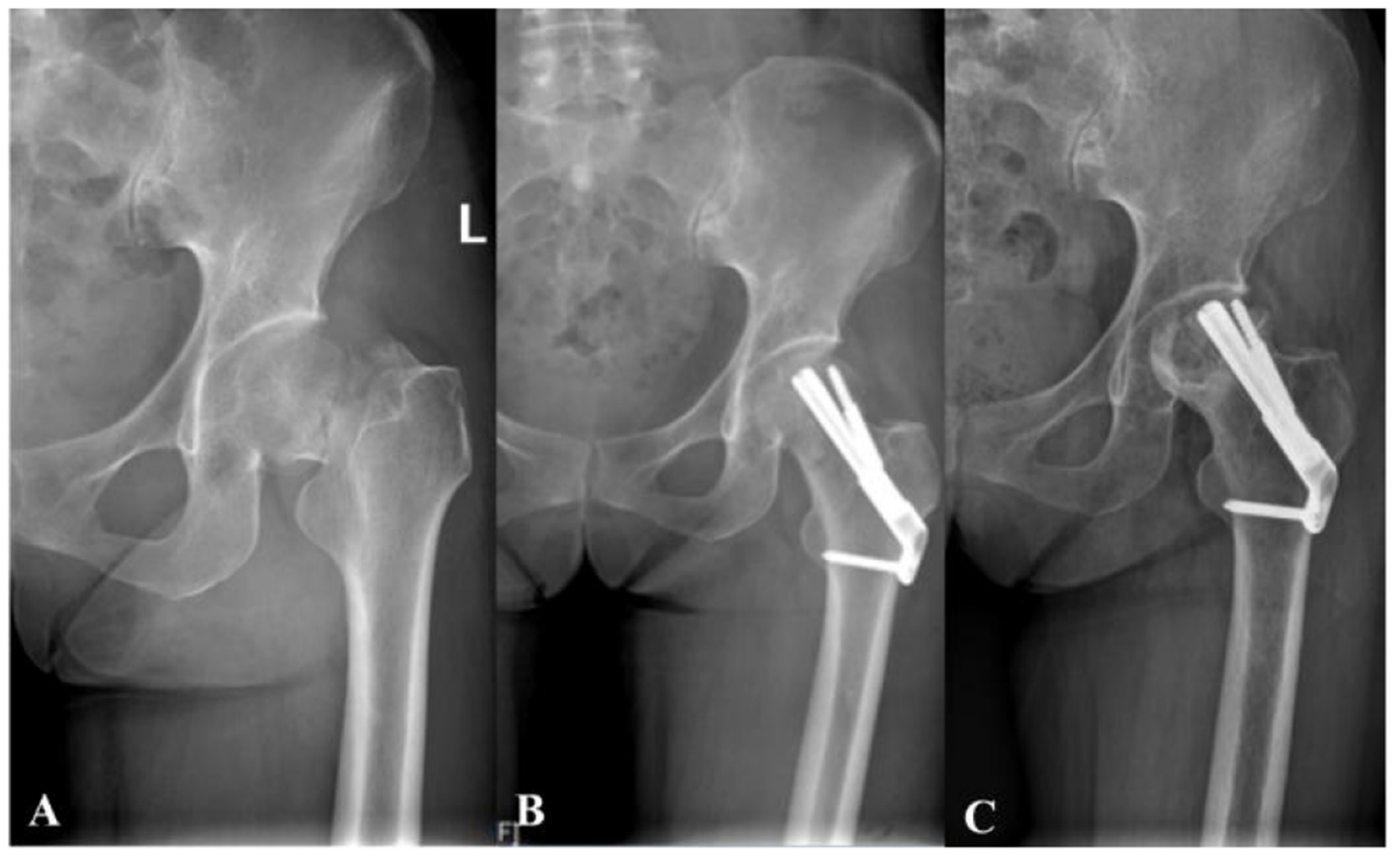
A 38-year-old female with femoral neck fracture. (A) Preoperative radiograph shows the initial fracture pattern. (B) Immediate postoperative radiograph demonstrates suboptimal positioning of the FNS dynamic screw in the superior region of the femoral head. (C) Final follow-up radiograph confirms the development of femoral head osteonecrosis with subsequent collapse.
